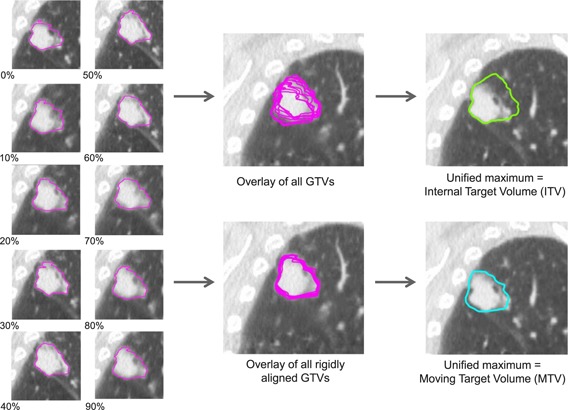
The ITV (green contour) is constructed as the unified maximum of the GTVs (magenta contours) of all ten 4DCT phases. The MTV (cyan contour) is constructed as the unified maximum of rigidly aligned GTVs, based on their center of volume. The CT images in the center column show the reference phase (peak‐exhale). The MTV is shown for a single phase only and is shifted for all other phases. The ITV is constant for all phases. The 4DCT data correspond to patient 3.
